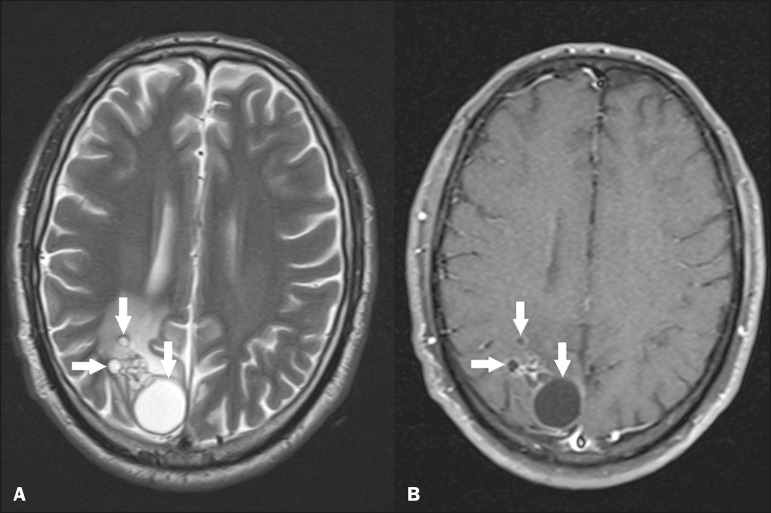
Alveolar echinococcosis. Axial T2-weighted MRI (A) and contrast-enhanced axial T1-weighted MRI (B), showing multiple coalescent cyst-like lesions in the right occipital lobe, characterized by a signal similar to that of the CSF in the T2-weighted sequence and peripheral contrast enhancement (arrows).
